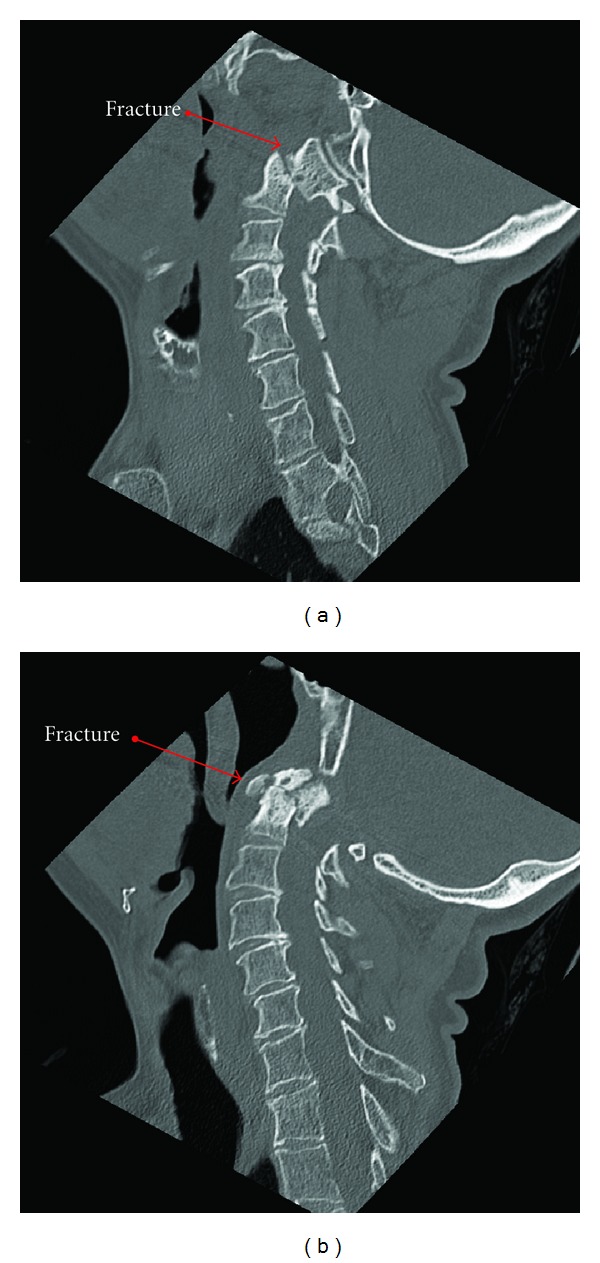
It shows noncontrasted MRI with cervical spine fracture.
